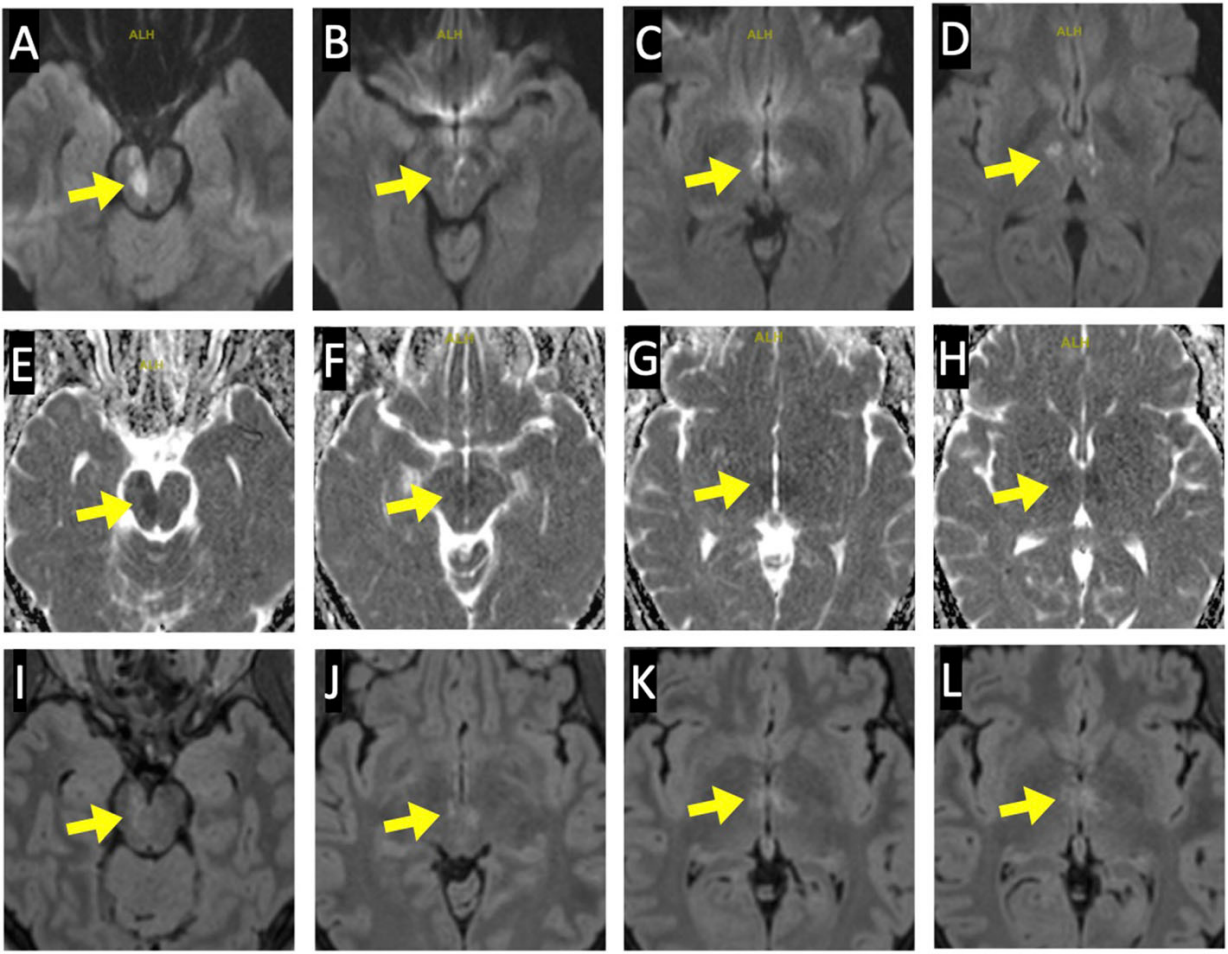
MRI findings of acute infarction in bilateral thalami and midbrain. Axial magnetic resonance images 1 day post-stroke demonstrating acute infarcts of the thalami and midbrain bilaterally (yellow arrow) as confirmed with increased signal on diffusion weighted imaging (a-d), corresponding signal drop out on apparent diffusion coefficient map (e-h), and hyperintensity on fluid attenuation inversion recovery sequences (i-l). For each row of images, axial slices are arranged caudally to rostrally
